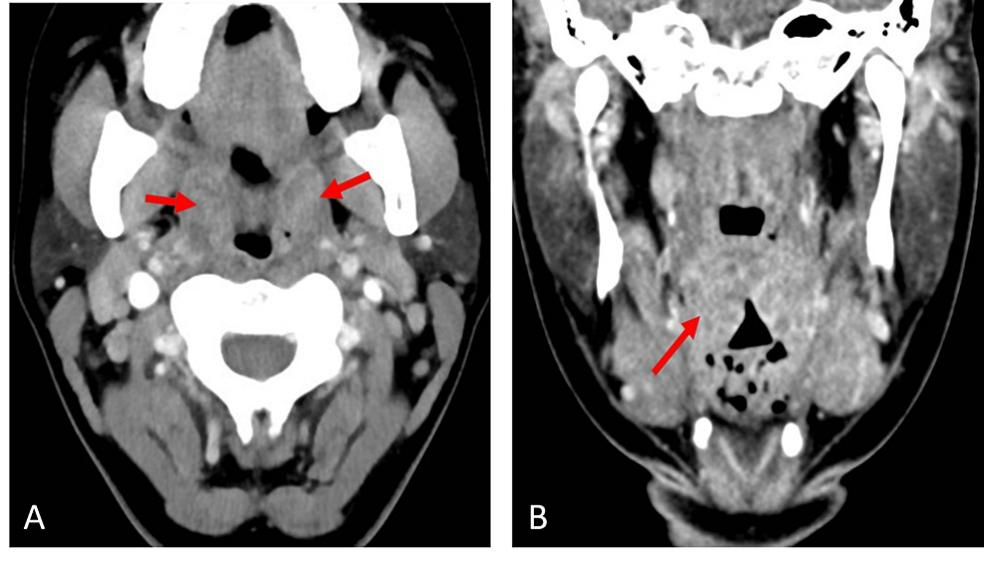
Tonsillitis Axial (A) and Coronal (B) View CT with contrast images demonstrate typical findings of tonsillitis to include bilateral tonsillar enlargement with striated internal enhancement (arrows) consistent with early intratonsillar phlegmonous changes. This scan is negative for a well-defined peripherally enhancing abscess.
